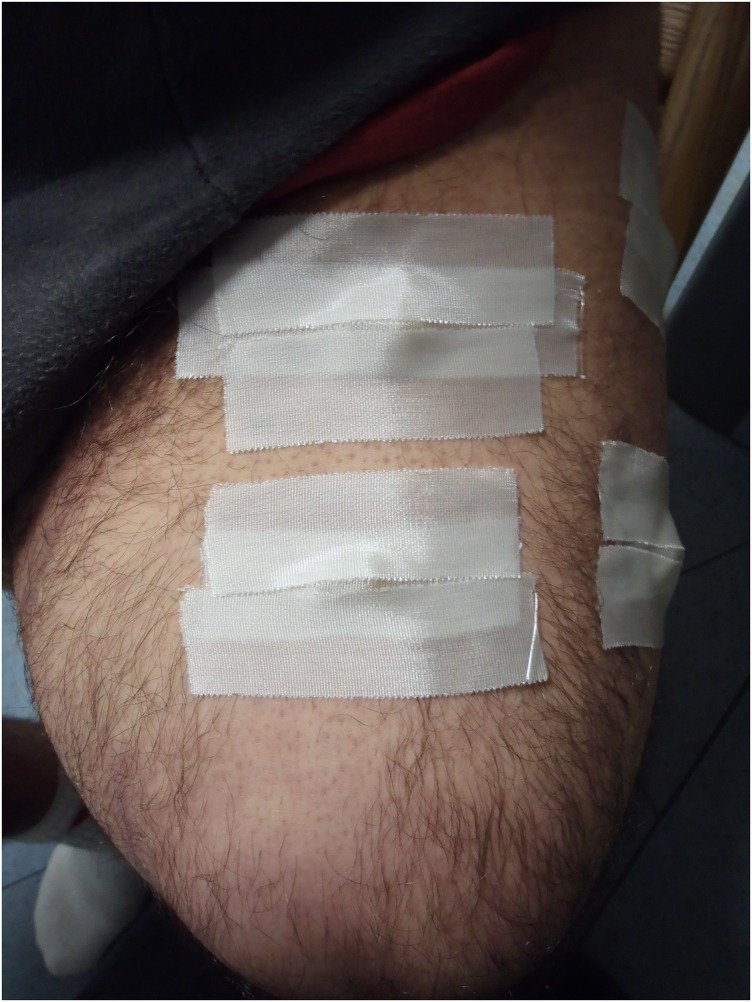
MRI markers correlating with locations of the ultrasound transducers on the stump.
